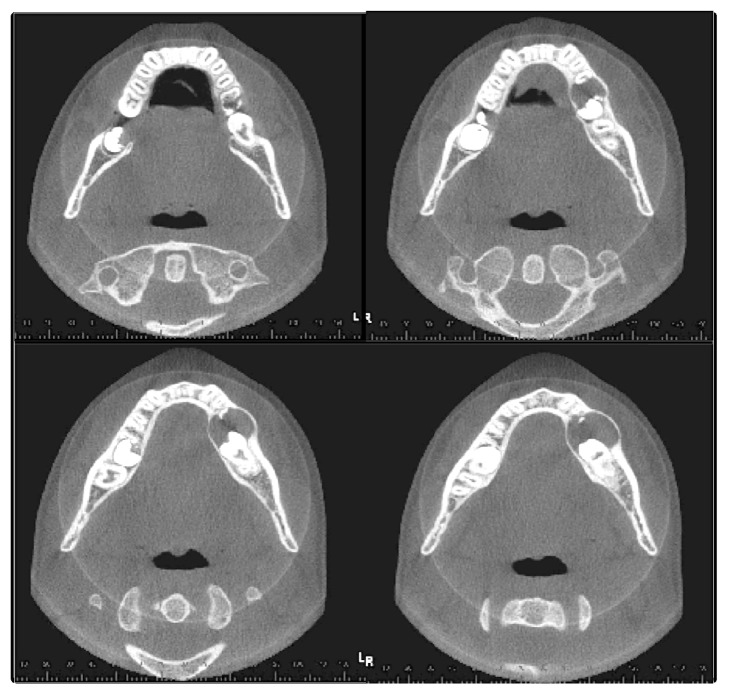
CBCT image, axial sections, showing bulging of osseous cortices in the region of the left permanent mandibular second molar.
